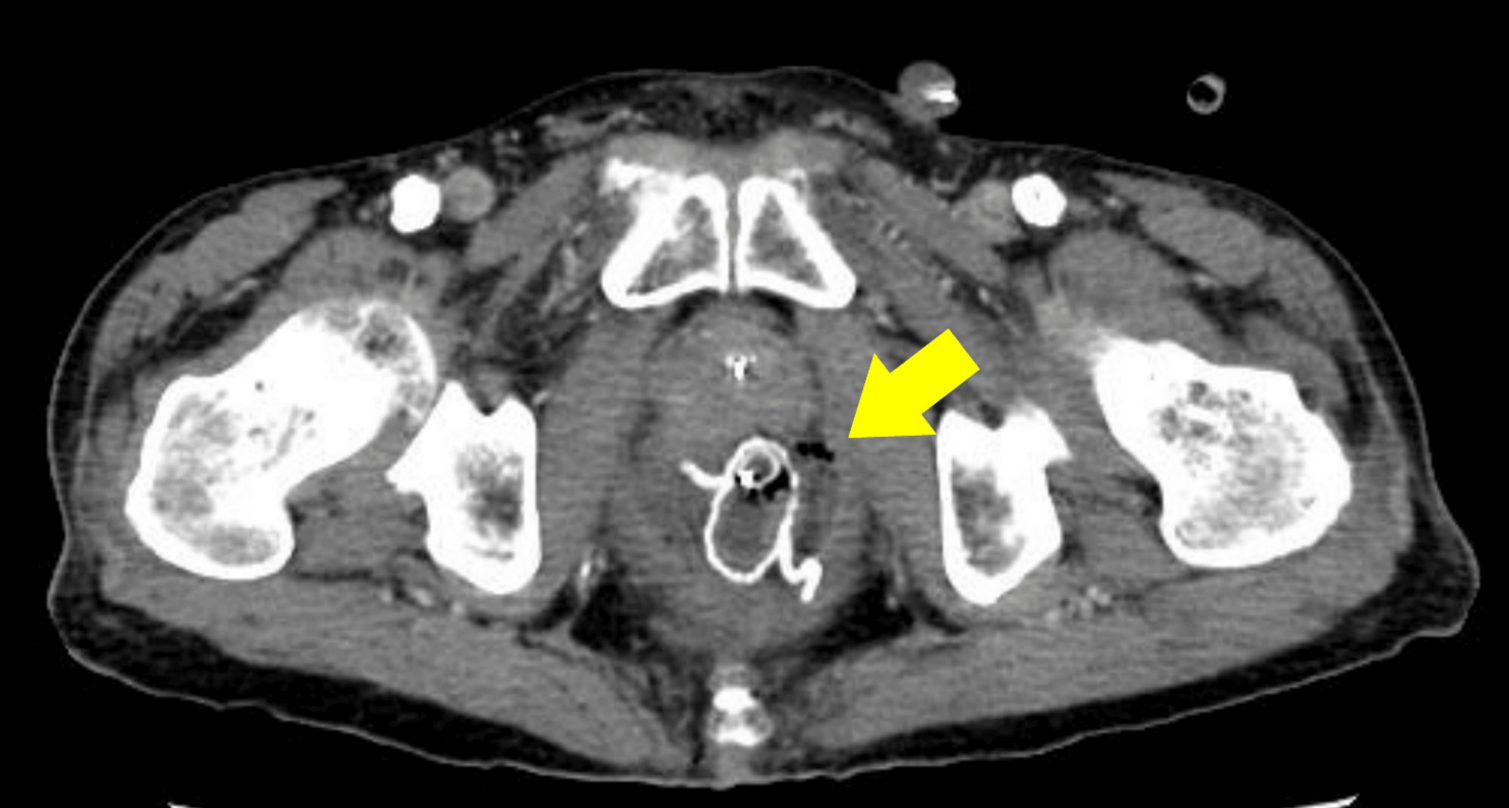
Contrast-enhanced CT. Staple line continuity at the anastomosis is preserved, but perianastomotic fluid and free air are present (yellow arrow).
